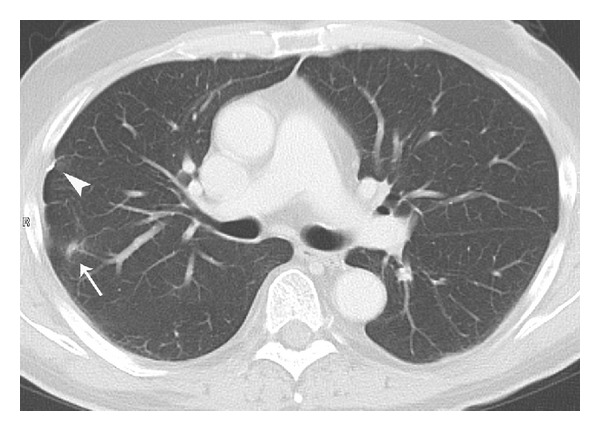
CT scan of the chest five months after the first application of chemotherapy. Small scar in the right upper lobe (white arrow) and small pleural calcification (white arrowhead) as residual lesions of the inflammatory process.
